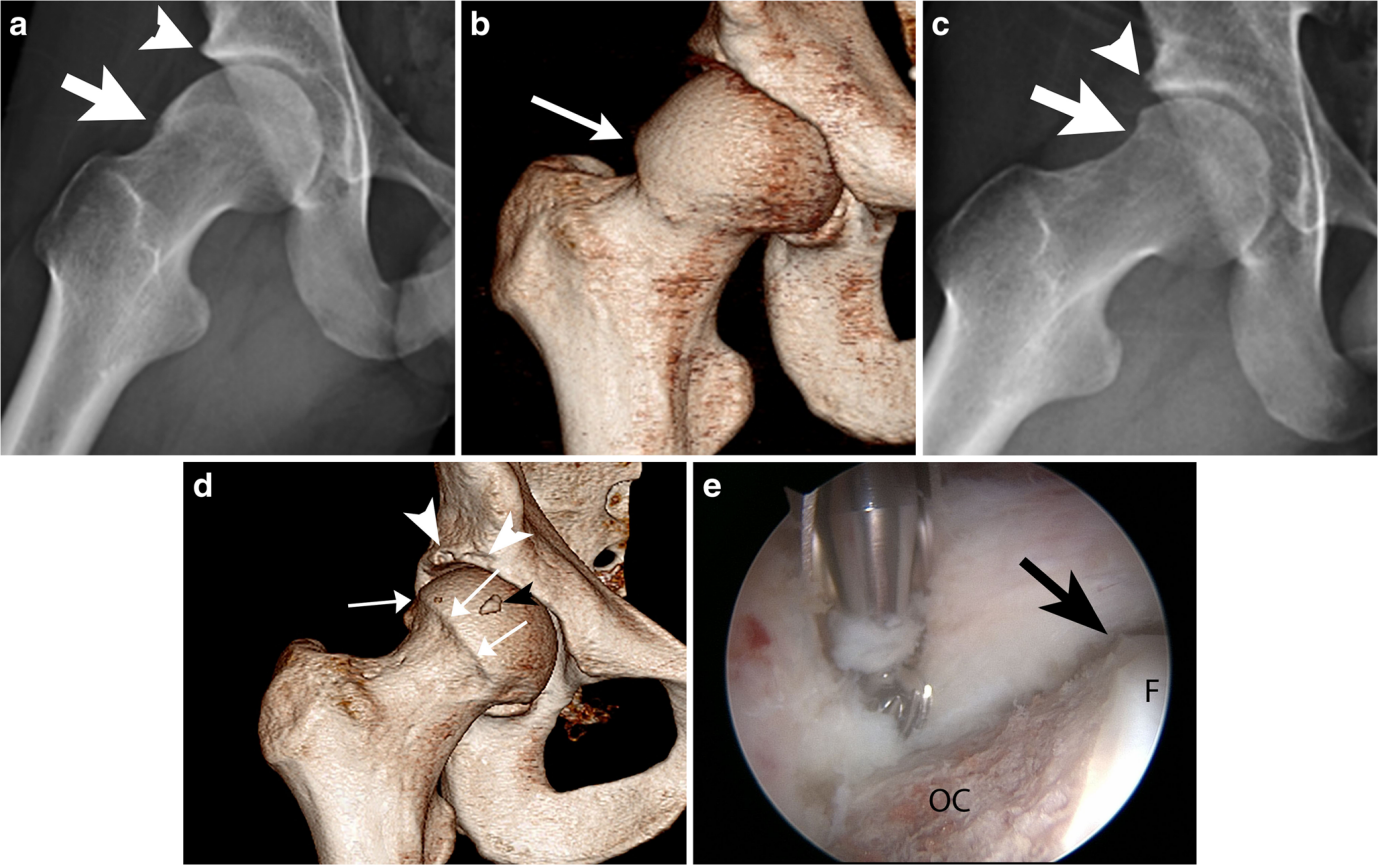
Cam lesion before and after surgery in a 20-year-old man. a Preoperative frog leg lateral view shows the anterolateral femoral bony prominence (*arrow*) known as a cam lesion. There is also mild prominence of the anterosuperior acetabular rim (*arrowhead*). b Preoperative 3D CT scan shows more fully the extent of the cam lesion at the anterior head–neck junction (*arrow*). c Postoperative frog leg lateral view shows osteochondroplasty site (*arrow*). Small focal lucency at the superolateral margin of the acetabulum (*arrowhead*) is the site of the “rim trim” and radiolucent suture anchor placed for labral repair. d Postoperative 3D CT scan shows an abrupt contour defect (*arrows*) at the site of surgical resection. This contour change can be mistaken by the unwary for an osteophyte. The “rim trim” and the site of labral reattachment (*white arrowheads*) are also visible. There is a small focus of heterotopic ossification (*black arrowhead*). e Arthroscopic image shows osteochondroplasty site (*OC*).* Arrow* points to the margin of osteochondroplasty.* F* femoral head
